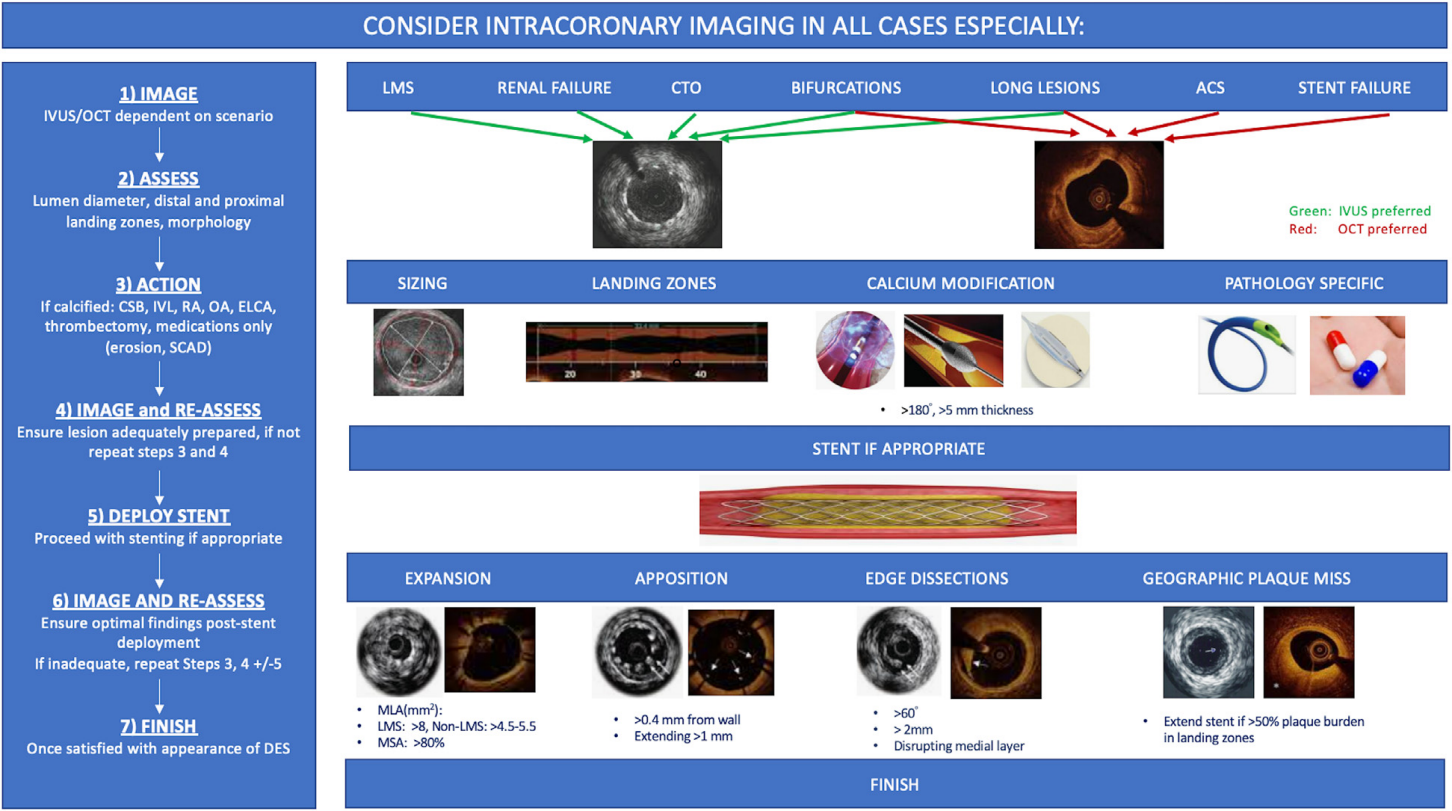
Algorithm for the use of intracoronary imaging. ACS, acute coronary syndrome; CSB, cutting/scoring balloon; CTO, chronic total occlusion; DES, drug-eluting stent; ELCA, excimer laser coronary angioplasty; IVL, intravascular lithotripsy; IVUS, intravascular ultrasound; LMS, left main stem; MLA, minimal luminal area; MSA, minimal stent area; OA, orbital atherectomy; OCT, optical coherence tomography; RA, rotational atherectomy; SCAD, spontaneous coronary artery dissection.
